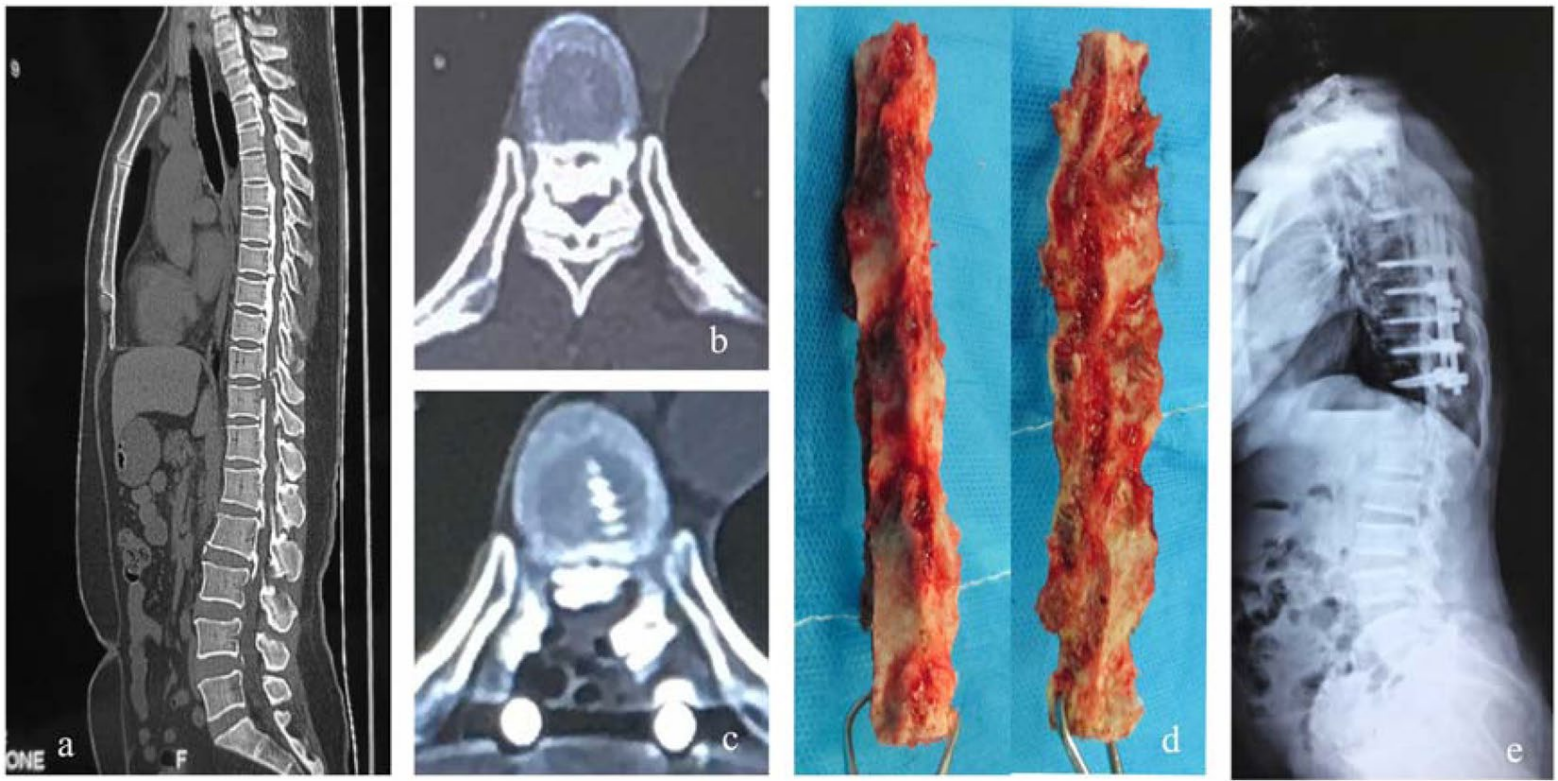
Utility of ultrasonic device in thoracic ossified posterior longitudinal ligament (OPLL) complicated with ossification of the ligamentum flavum (OLF) 45-year old female patient presented with weakness and numbness of the lower extremities, she also had urinary dysfunction and was unable to ambulate (JOA, 5). Figure 2a: computed tomography shows continuous OPLL ranging from cervical to lumbar levels; Fig. 2b and c demonstrate severe impingement of cord by concomitant OPLL and OLF and posterior shift of the spinal cord after operation; Fig. 2d shows the resected laminae by posterior laminectomy with instrumented fusion (T6–11); Fig. 2e demonstrates the postoperative radiograph of the spine. She experienced improvement rate of 41.7% without complications 12 months postoperatively.
